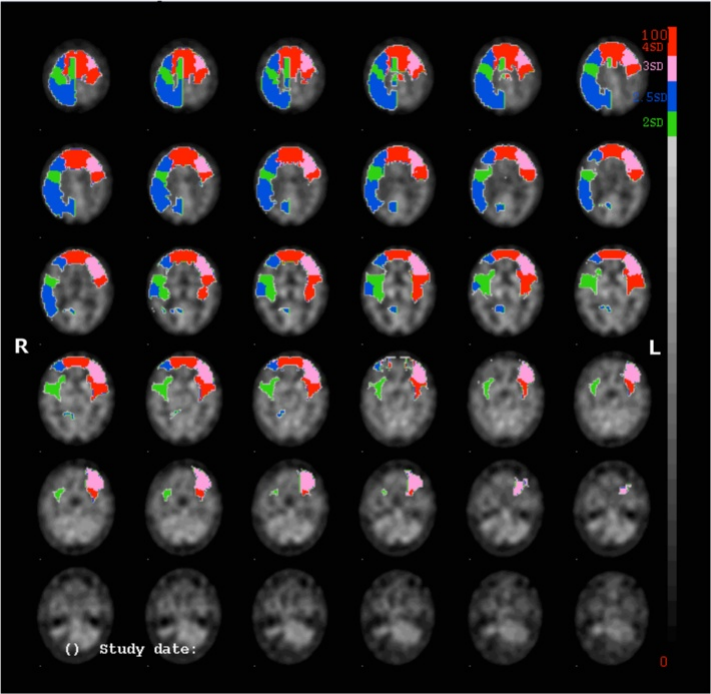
SPECT scan of the brain showing a bilateral hypoperfusion in the prefrontal cortex more pronounced on the left than on the right side. A significant decrease of perfusion is also found in the motor cortex and the right parietal cortex. Left and right are as indicated in the figure
